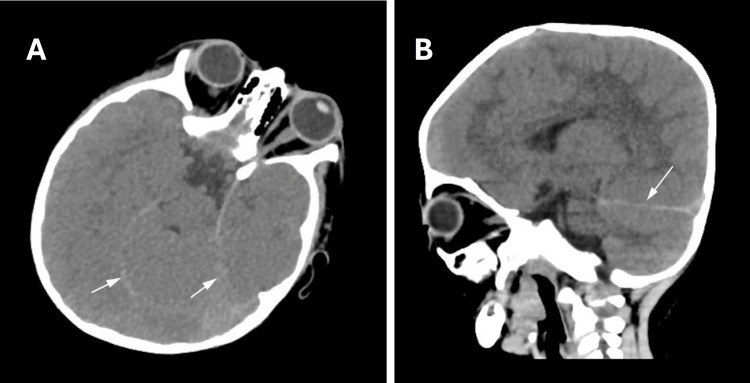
Non-contrast computed tomography of the brain on admission. (A) Axial image demonstrating bilateral linear hyperdensities along the tentorial margins (white arrows). (B) Sagittal reconstruction demonstrating corresponding tentorial hyperdensity (white arrow). No parenchymal hemorrhage, mass effect, midline shift, or hydrocephalus was identified. These appearances initially raised concern for subarachnoid hemorrhage and prompted advanced venographic imaging.
